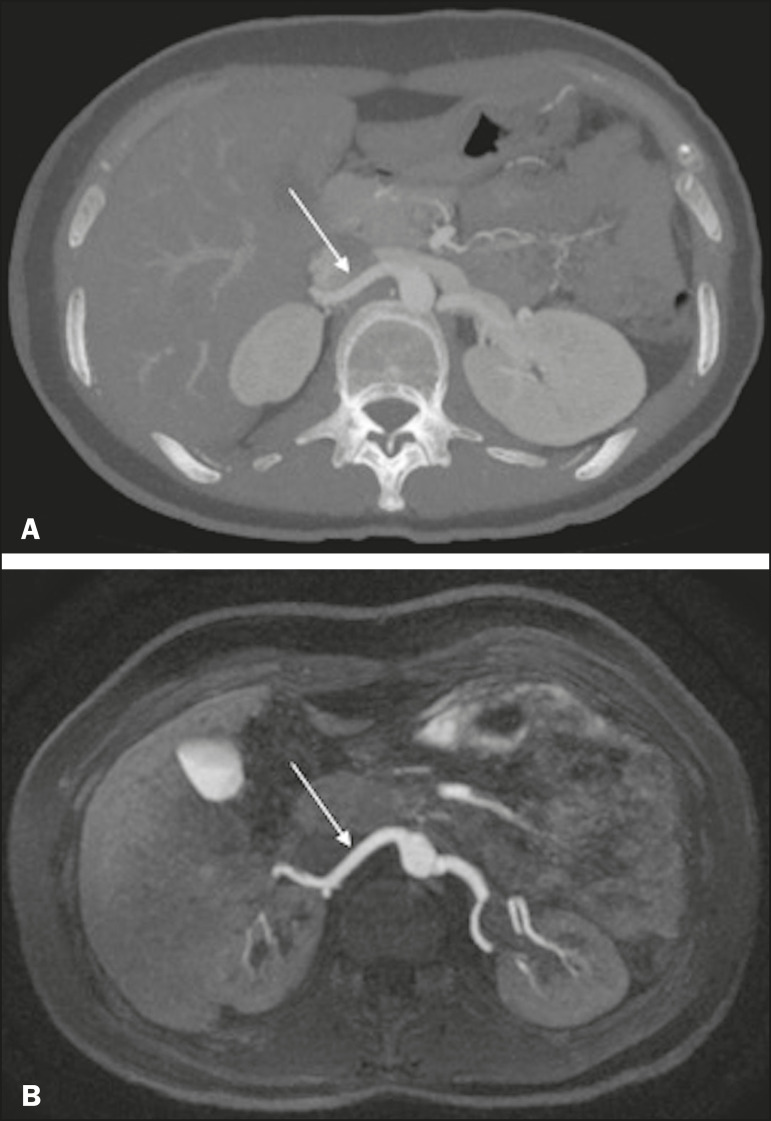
Concordance between the two methods to evaluate the quality of the images. Axial CTA (A) and axial unenhanced MRA (B) images of the proximalsegment of the right renal artery (arrows) considered to be of diagnostic quality.
